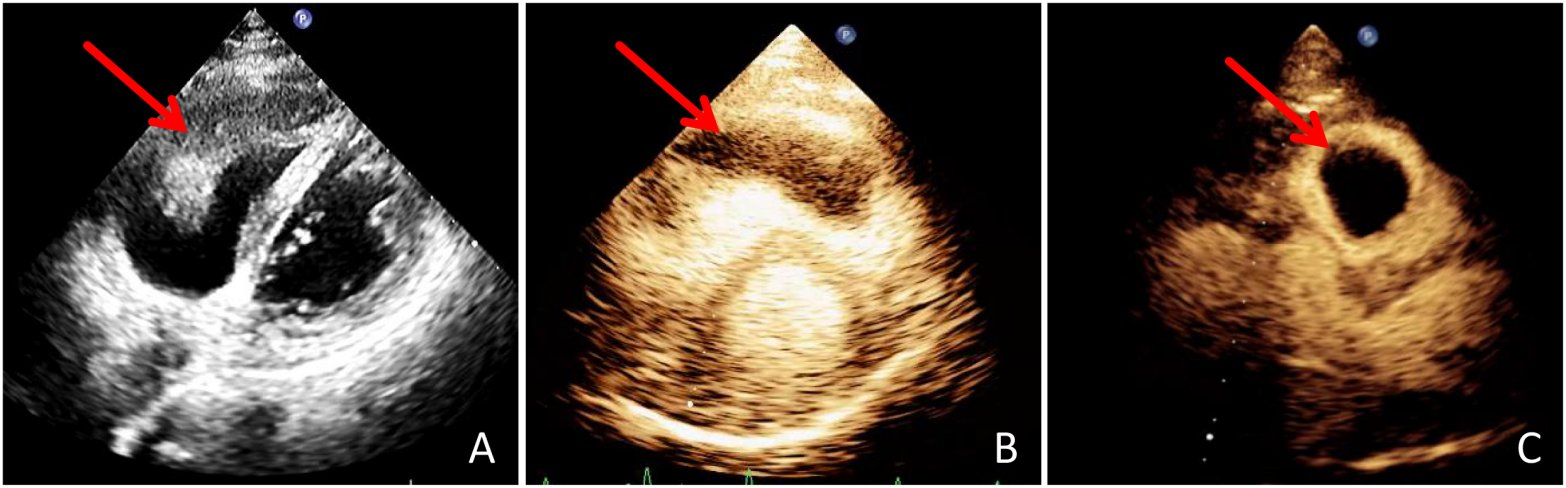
Echocardiography (A) reveals an isoechoic mass within the right ventricular cavity that is connected to the right ventricular wall and extends into the pulmonary artery(arrow). MCE (B) shows no significant contrast filling within the mass in the right ventricular cavity (arrow). After rotating the probe 90 degrees, the cross-sectional view (C) demonstrates no significant contrast filling within the mass in the right ventricular cavity (arrow).
